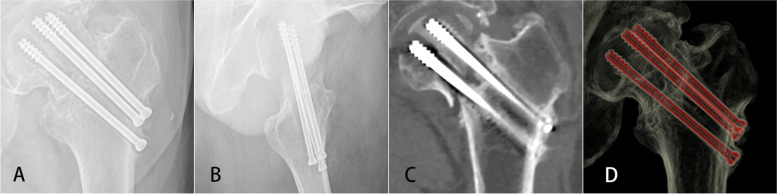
A case of unhealed femoral neck fracture. A Coronal X-ray, B sagittal X-ray, C coronal CT, D sagittal CT
